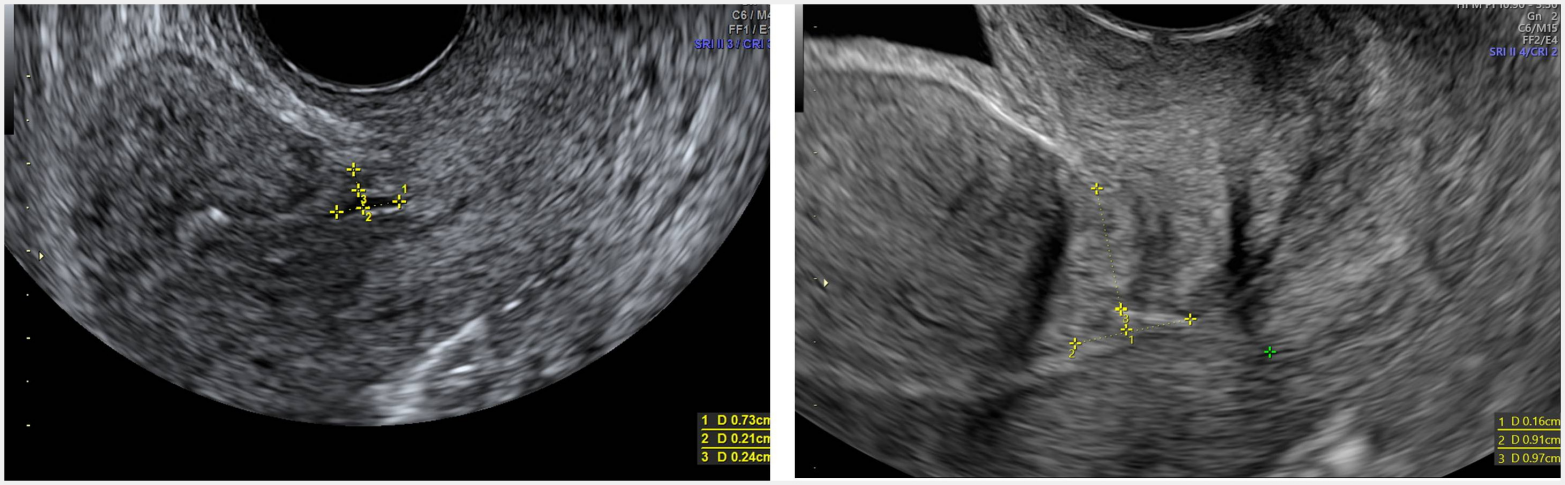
Preoperative and postoperative transvaginal ultrasound scan of cesarean scar defect (CSD). In the sagittal scan, calipers showed measurements of residual myometrial thickness (RMT), base and height diameter before surgery (left image) and at 4‐month follow up (right image).
